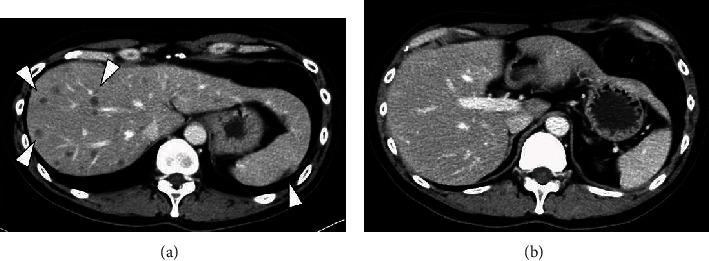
Liver metastases before and after the combination chemotherapy of cisplatin and etoposide: (a) computed tomography showed multiple liver metastases (arrows); (b) liver metastases had achieved complete response in a patient who received combination chemotherapy of cisplatin and etoposide.
